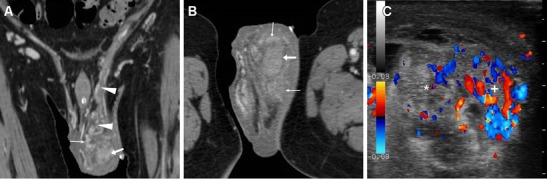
Acute epididymo-orchitis in a 47-year-old Sri Lankan man with multiple myeloma on bortezomib therapy, suffering from fever and acute scrotal pain, tenderness and induration. Contrast-enhanced CT (a, b) performed to investigate impending urosepsis depicted a thickened engorged left spermatic cord, with inhomogeneous vascularisation of the ipsilateral epididymis (*thin arrows*) and testis (*arrows*). Note catheter (in a), thickened and increased oedematous attenuation of the scrotal skin and external tunicae. CD-US (c) revealed hypervascularisation of the epididymis (+). Unresponsive to antibiotics, *Klebsiella pneumoniae* ultimately required orchiectomy
